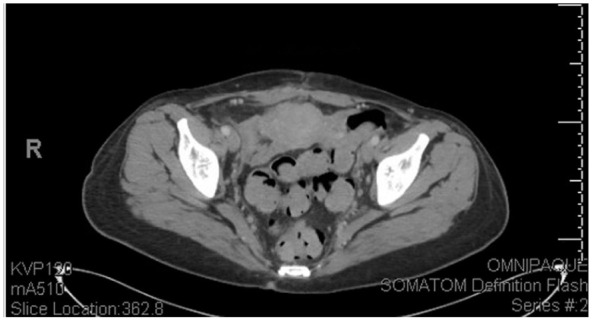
CT Abdomen Pelvis 26 days post-discharge: Interval resolution of right complex fluid collection now heterogenous granulation tissue and inflammation in the right adnexa but with no drainable fluid collection. New 3.3cm cm × 3.3 cm thin-walled cyst in the left adnexa associated with the left ovary. No pelvic free fluid. No inguinal or pelvic adenopathy.
